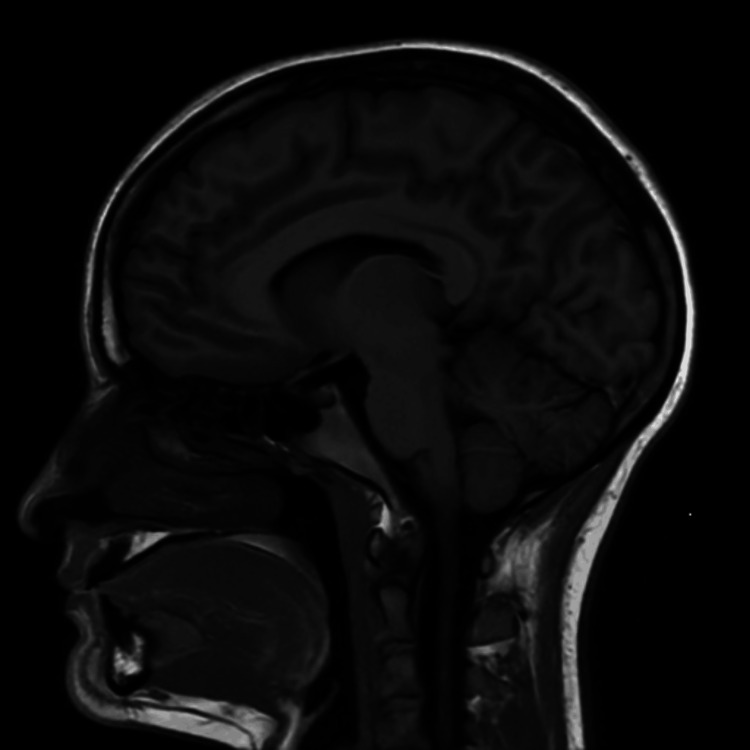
MRI of the brain without contrast showing normal cerebellar tonsils without ectopia or bulging through the foramen magnum.
